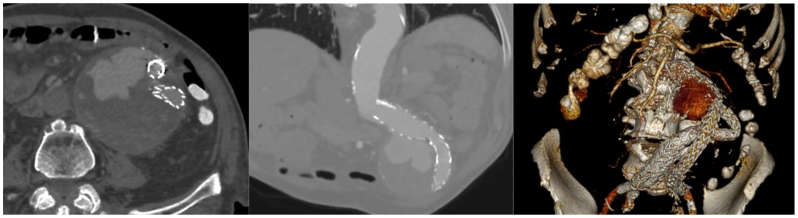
Computed tomography angiography (CTA) scan demonstrated a type Ib endoleak diagnosis: maximum intesity projection (MIP) and surface shaded display (SSD) show the contrast dine in the aneurismal sac.
